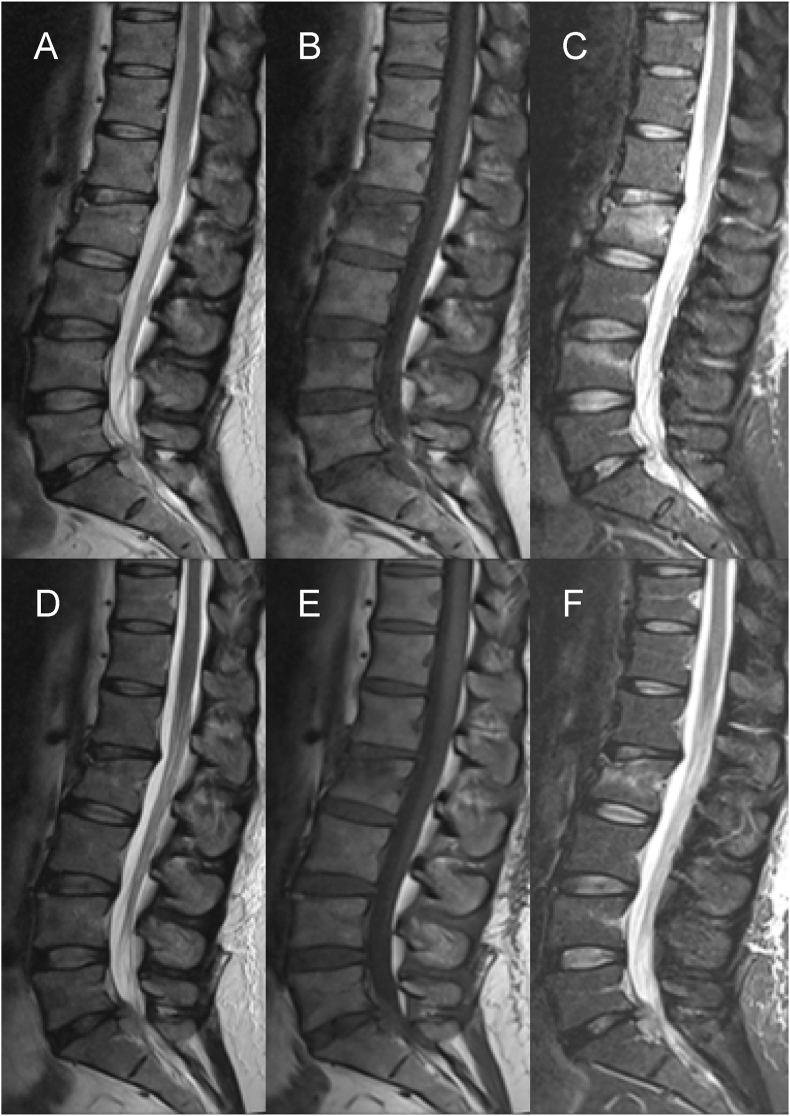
(MRI-after the trauma: A-T2 weight, B-T1 weight, and C-stir) The remaining disc tissue in the L5/S1 disc space and the giant sequester showed slightly weakened hyperintensity on T2 signal compared to the cerebrospinal fluid, as well as homogeneous hypointensity on T1 signal like the spinal cord. The STIR-intensities of sequestration and CSF were almost the same. The MRI findings at the follow-up (D-T2 weight, E-T1 weight, and F-stir) showed a further decrease in T2 signal intensity (D) compared to the MRI obtained one month prior (A). The difference in T2-intensity (D) and STIR-intensity (F) between the sequestration and liquor signal was more significant (D). The volume of intraspinal sequestration slightly decreased.
